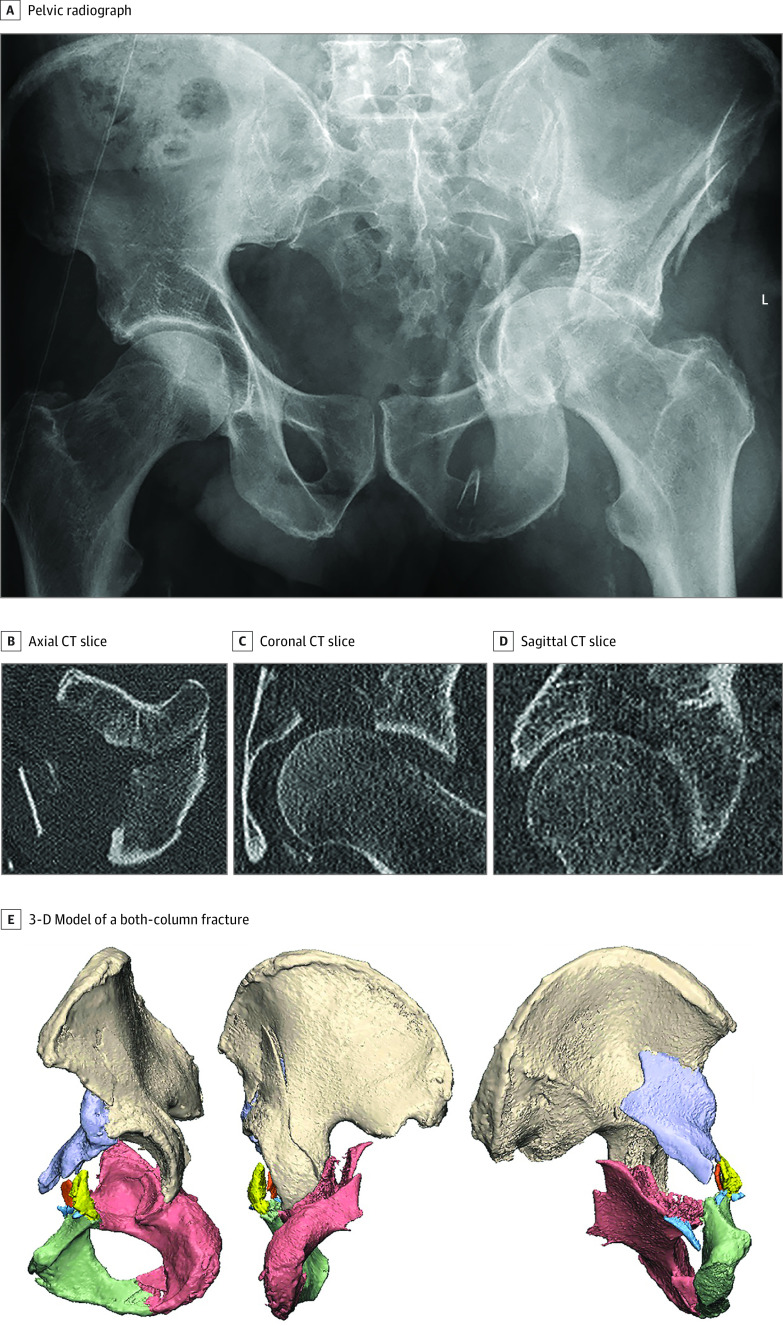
Preoperative Situation Pelvic radiograph, CT-scan (axial, coronal, sagittal CT slices) and 3-D model from patient 5, who had fallen from a height and sustained an associated-type both-column fracture. The fracture caused medial protrusion of the femoral head and severe displacement of the anterior as well as the posterior column. This patient was treated with patient-specific osteosynthesis plates.
